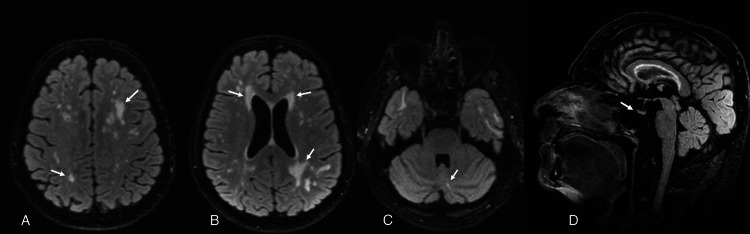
Brain MRI of Patient 3 (A) Axial FLAIR image showing bilateral frontoparietal hyperintensities sparing the U-fibers; (B) axial FLAIR images showing periventricular and deep white matter hyperintensities; (C) axial FLAIR image showing tiny left cerebellar white matter hyperintensity; (D) sagittal FLAIR image demonstrating small pituitary gland.
